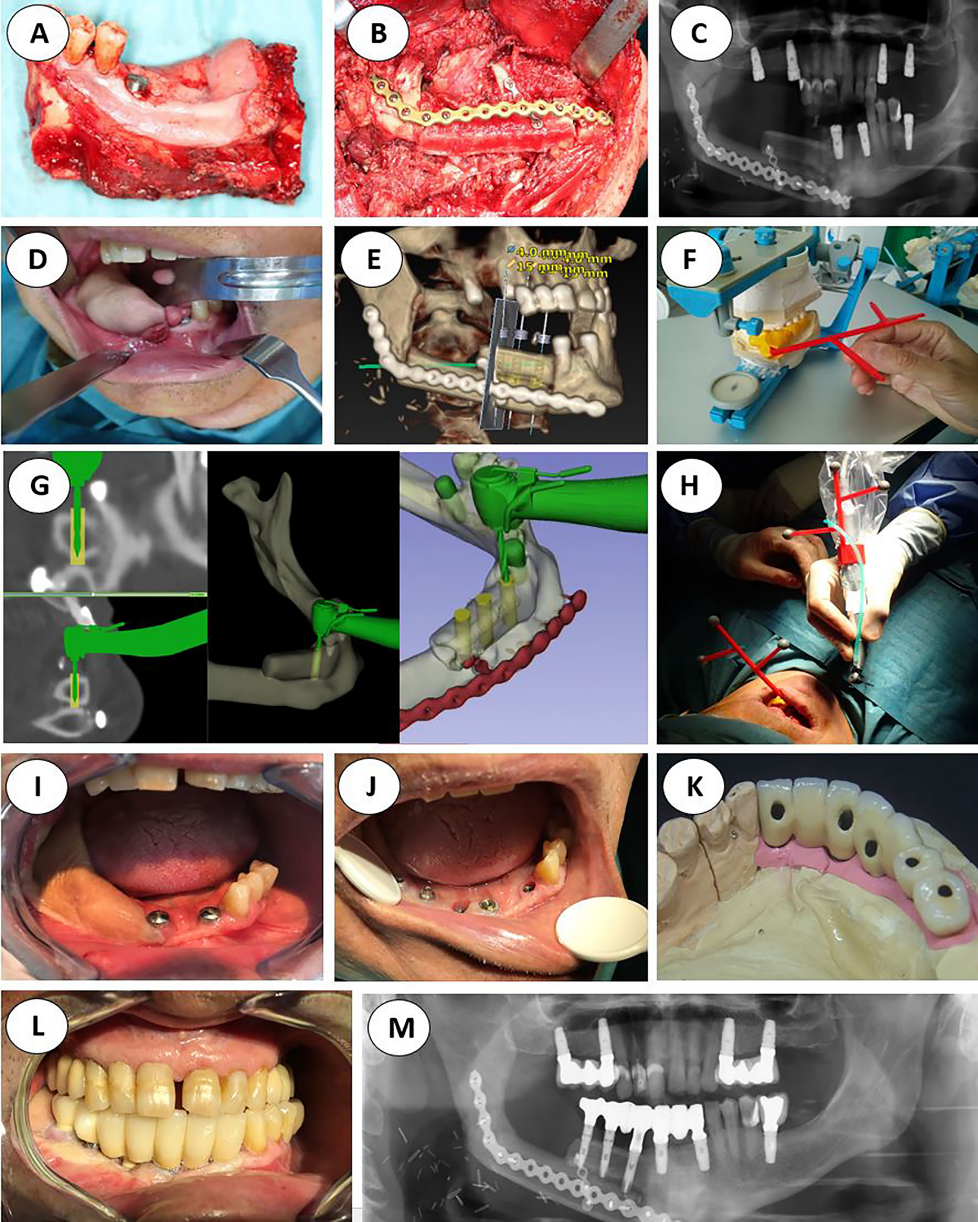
Initial protocol example, from the ablative surgery to the final orthopantomogram (A) Right segmental mandibulectomy. (B) Double-barrel fibula flap in place. (C) Postoperative orthopantomography. (D) Redundant fibula skin paddle. (E) Implant placement virtual surgical planning with Nobel Clinician-DivX software. (F) Teeth supported silicone jig holding the 3D-printed dynamic reference frame. (G) Intraoperative screen view of the navigated handpiece and the real-time drilling trajectory. (H) Handpiece and patient’s optical markers ready for dynamic navigation. (I) Still redundant skin paddle after implant surgery. (J) Intraoral view after vestibuloplasty and implant second phase. (K) Screw retained porcelain fused to metal fixed prosthesis lingual view. (L) Final occlusion. (M) Final panorex with the prosthesis in place.
